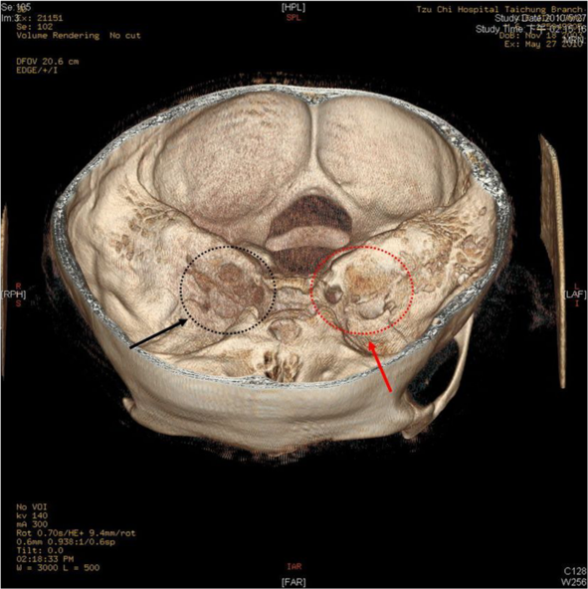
Reconstruction in brain HRCT showed multiple bony destructions at the right side (*black arrow*) compared to the left side (*red arrow*)
